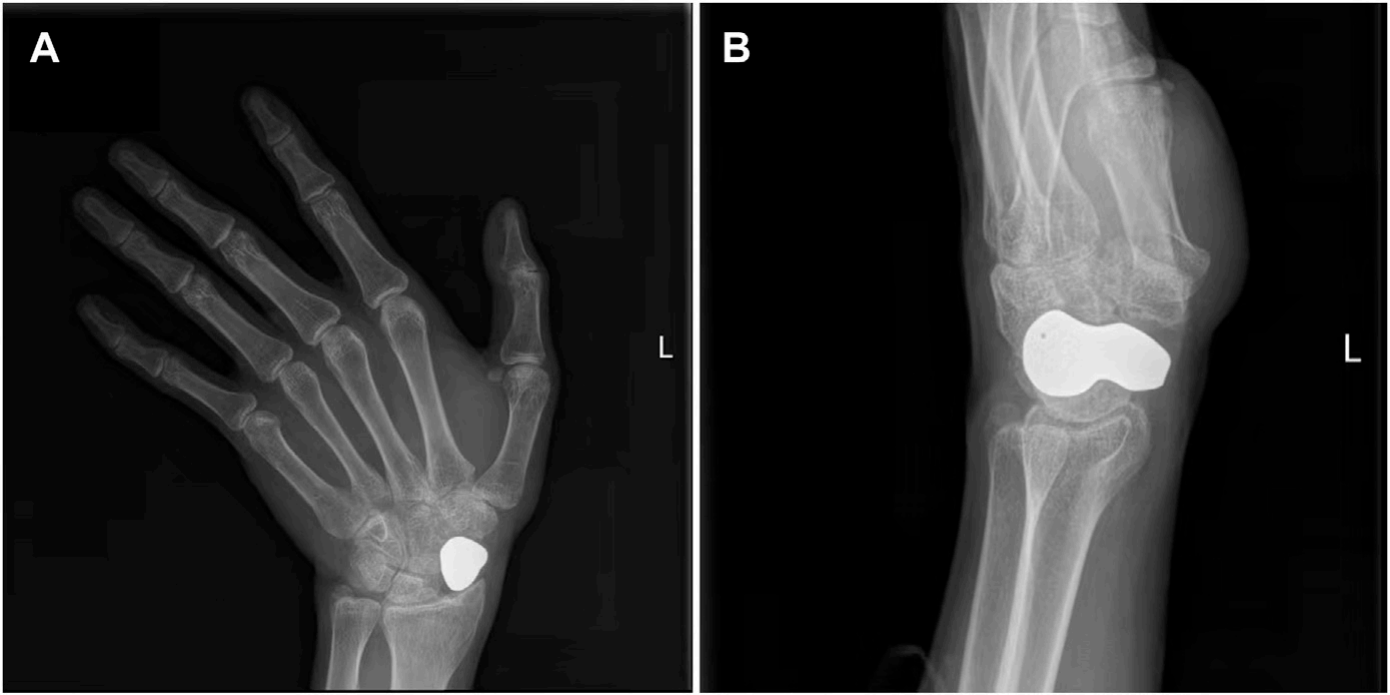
Typical case. (A) Anteroposterior radiograph of the scaphoid prosthesis 20 months after surgery; (B) Lateral radiograph of the scaphoid prosthesis 20 months after surgery.
